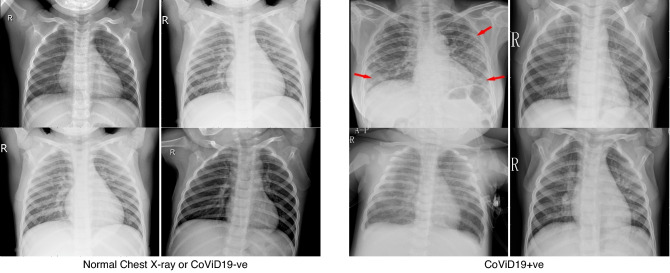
Chest X-ray of normal (CoViD19−ve) and CoViD19+ve.
